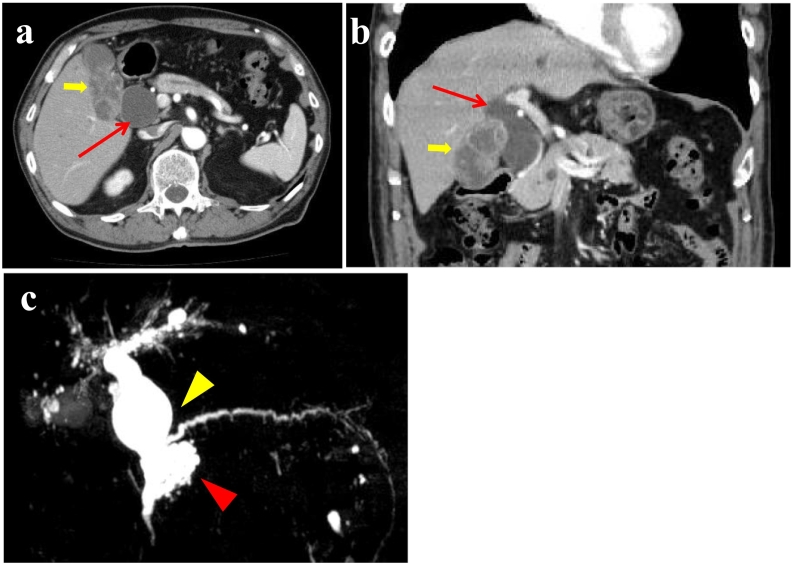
Preoperative CT and MRI. a-b A horizontal image (a) and a coronal image (b) of an enhanced CT scan showing enhanced wall thickness in the gallbladder (yellow arrow). The common bile duct was dilated up to 43 mm (red arrow). c A MRCP scan showed an anomalous junction of pancreaticobiliary tracts. Both the common bile duct and the intrahepatic bile duct are dilated (yellow arrowhead). The pancreatic uncinate process is occupied by a “bunch of grapes” lesion apart from main pancreatic duct suggesting the branch duct type IPMN (red arrowhead). (For interpretation of the references to colour in this figure legend, the reader is referred to the web version of this article.)
